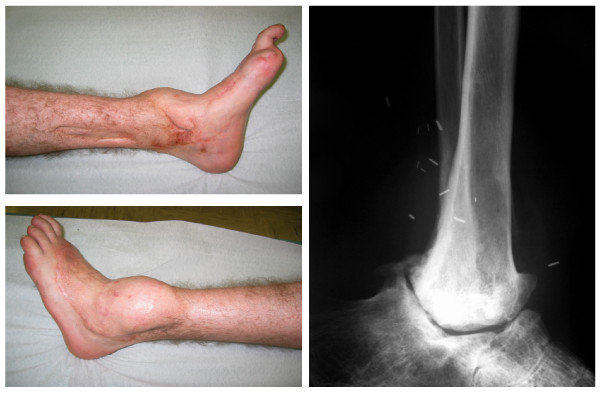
Clinical status of the lower leg at the 19-year follow-up examination. Medial and lateral views (left), and radiograph showing tibiocalcaneal pseudarthrosis (right).
